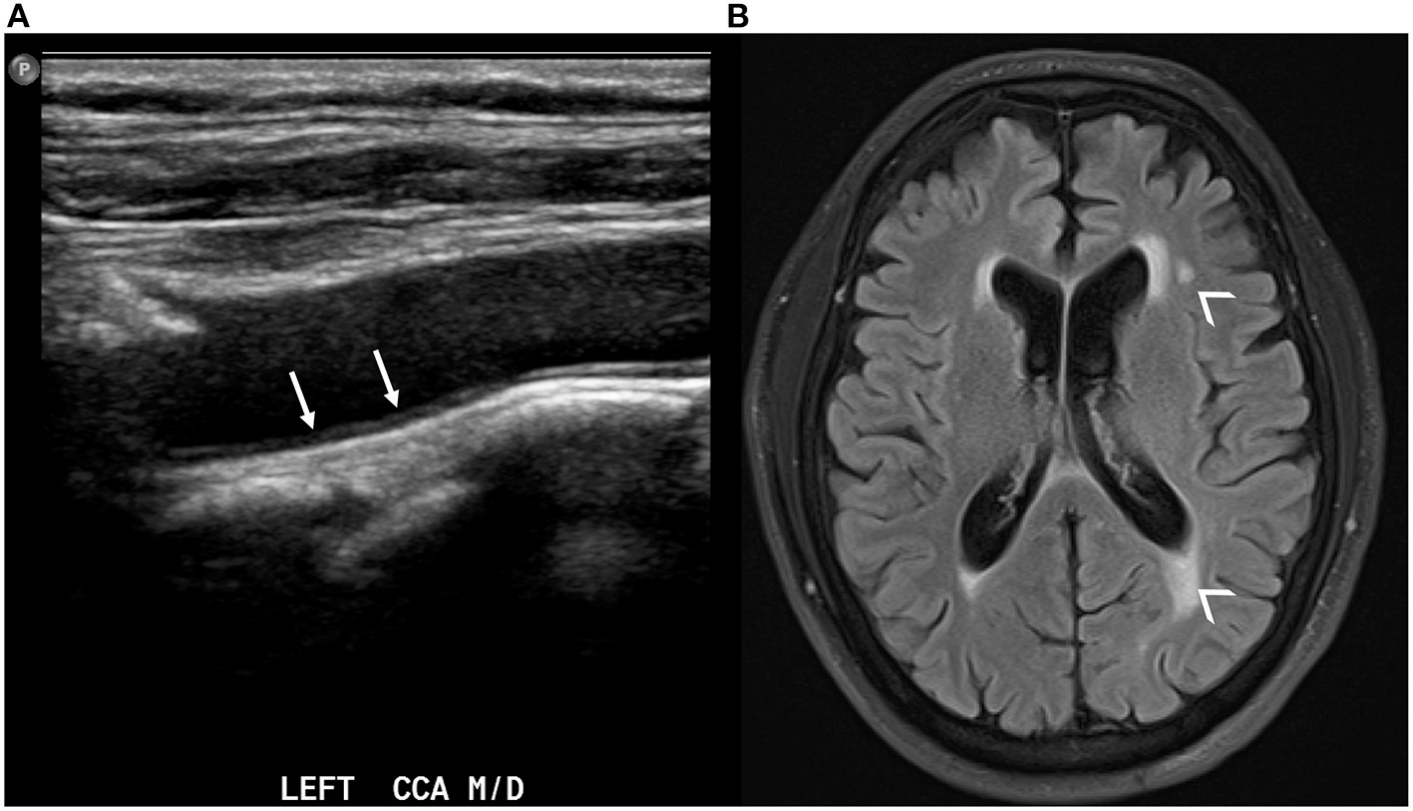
Carotid ultrasound demonstrating thickening of the intima-media in the left common carotid artery [(A), white arrows]. The same patient also has evidence of white matter hyperintensities in the periventricular white matter on axial T2 FLAIR brain MRI [(B), small arrowheads].
